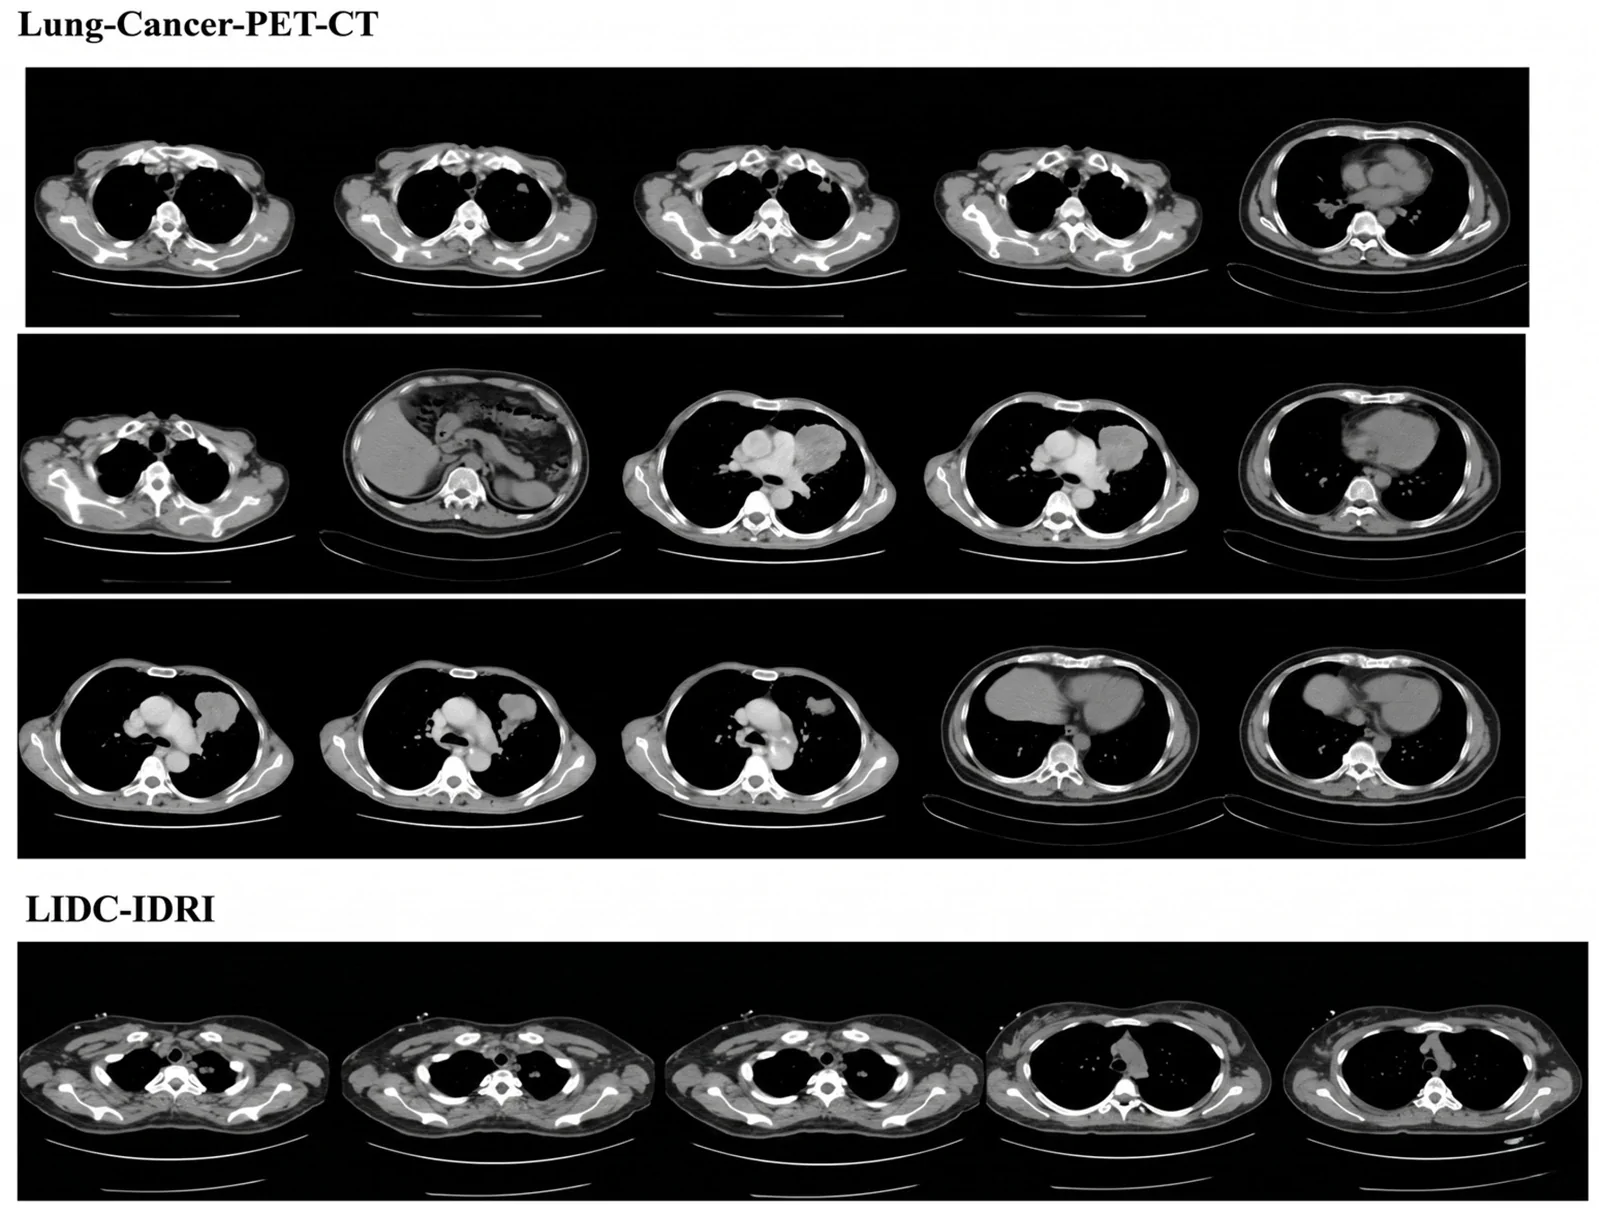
Example images from the LUNG-PET-CT-DX and LIDC-IDRI datasets used for training, internal validation, and external evaluation. The datasets include CT slices with diverse anatomical structures and pathological variations relevant to pulmonary nodule detection.
